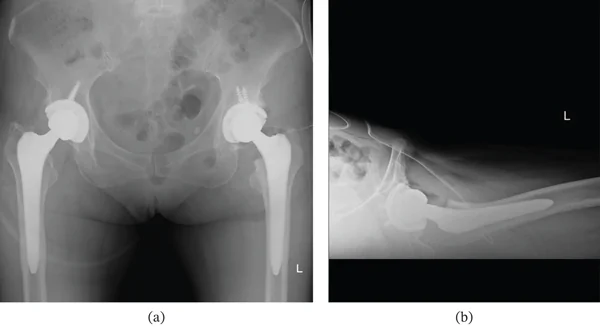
Findings on routine postoperative images obtained to assess for intraoperative and postoperative fractures as well as cup positioning after total hip arthroplasty. (a) Anteroposterior radiograph showing no evidence of stem subsidence or periprosthetic fracture. (b) Cross‐table lateral radiograph showing no evidence of stem subsidence or periprosthetic fracture.
